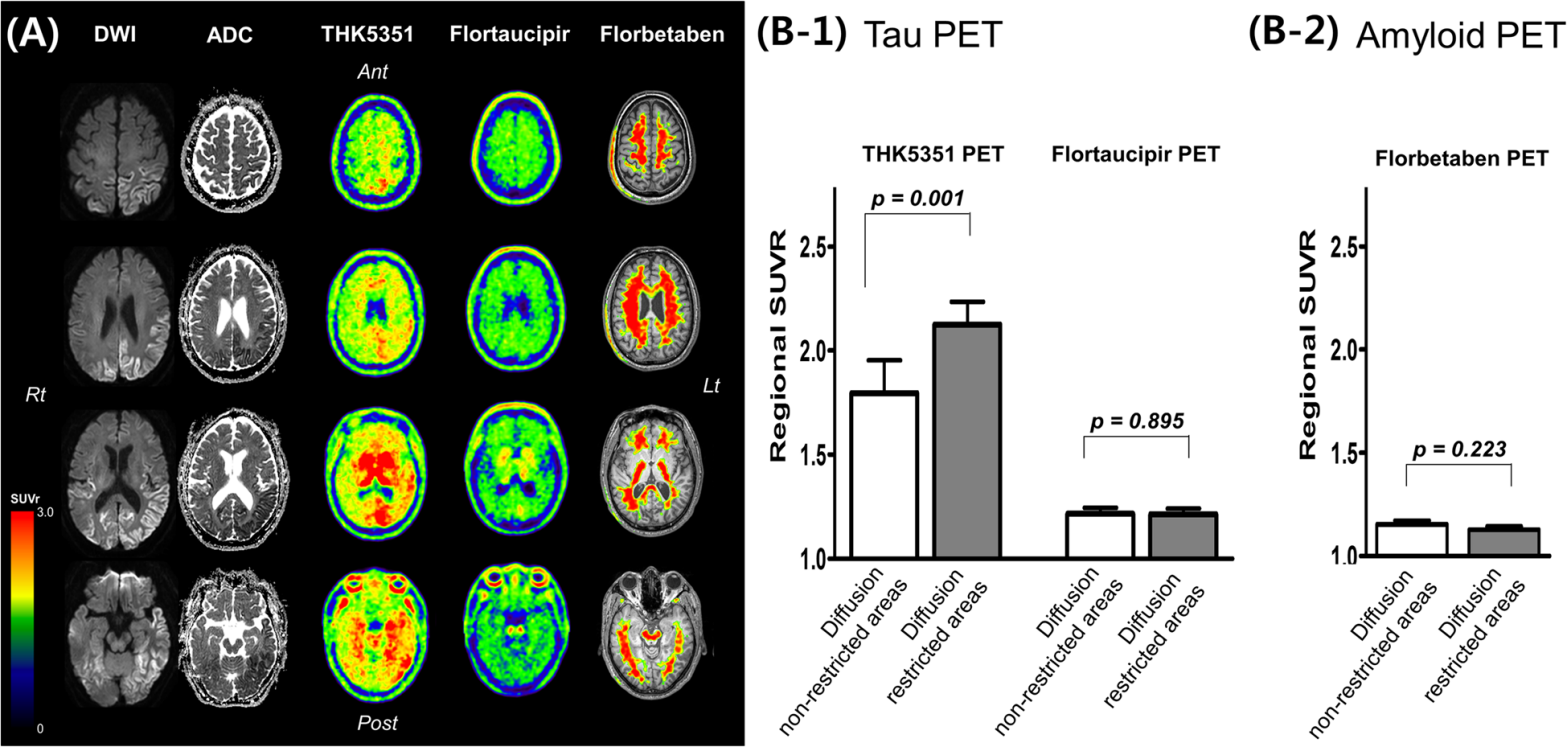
a Diffusion weighted images (DWI), apparent diffusion coefficient (ADC), tau (THK5351 and flortaucipir, and amyloid (florbetaben) PET images in a patient with sporadic Creutzfeldt-Jakob disease. b Regional standardized uptake value ratio (SUVR) of THK5351, flortaucipir, and florbetaben in diffusion non-restricted and restricted areas
